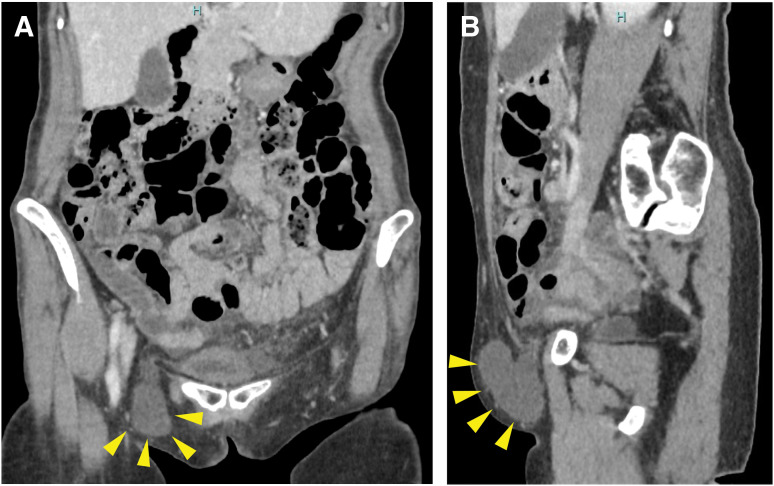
Contrast-enhanced CT of the abdomen. Showing a hydrocele in the groin, measuring 57.4 × 57.0 × 22.2 mm, medial to common femoral vessels and caudal to inguinal ligament (arrowheads). (A) Coronal section; (B) Sagittal section.
